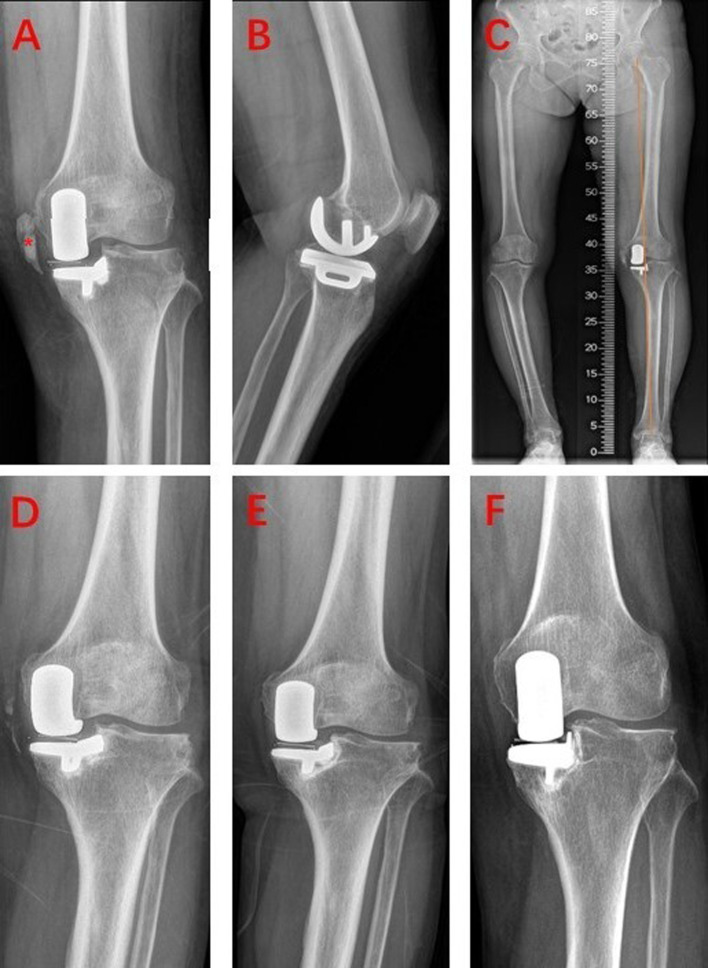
Postoperative x-ray during discharge and follow-up. (A–C) X-ray at discharge, *Pellegrini–Stieda lesion; (D) postoperative 3 months; (E) postoperative 9 months; (F) postoperative 3.5 years.
